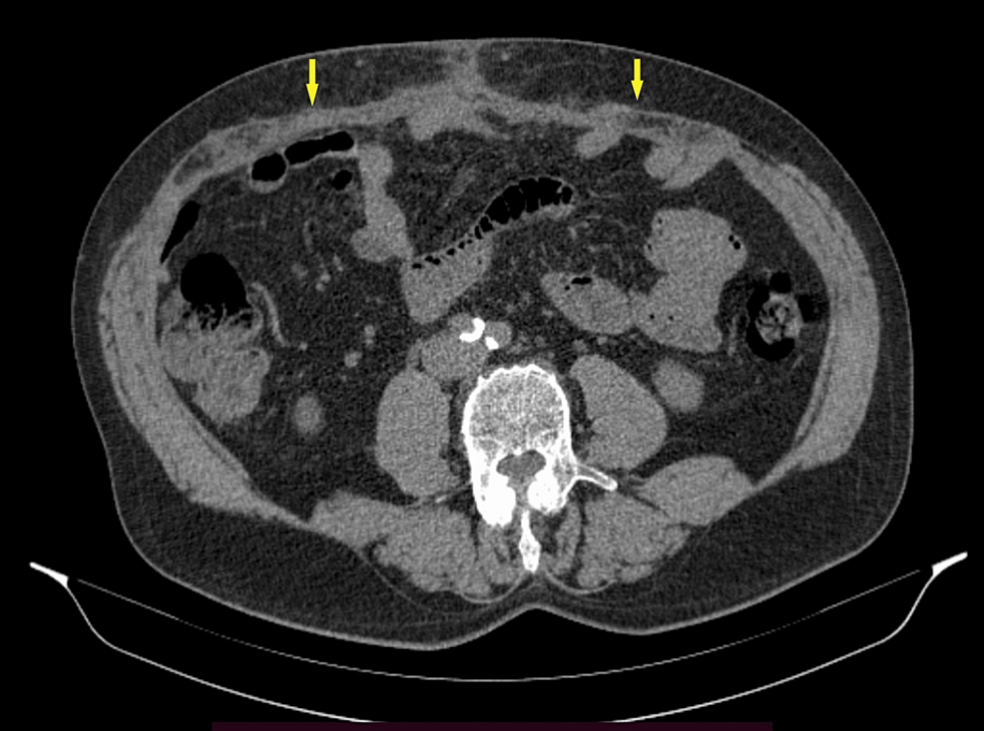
Postoperative control abdomen CT scan, 17 months after TAR, with restored abdominal wall continuity (arrows) CT: computed tomography; TAR: transversus abdominis muscle release
